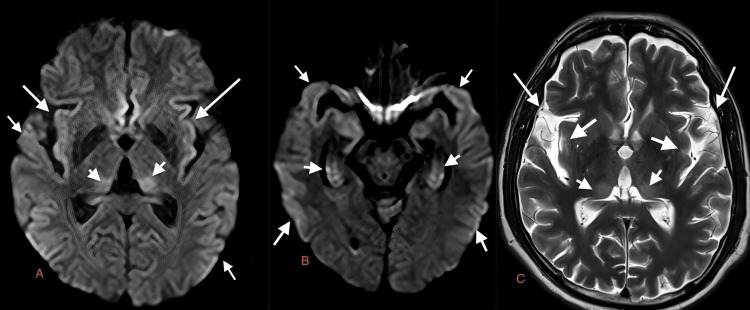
Case 6: Diffuse gyral areas of diffusion restriction are seen in both cerebral hemispheres on DWI (white arrows in A and B), predominantly in the temporal lobe also involving both hippocampi on day one of presentation in a 15-year-old male patient with dengue encephalitis. Areas of diffusion restriction are also seen in both thalami on DWI with corresponding hyperintensities on T2W images (white short arrows in A, B, and C, respectively). T2W: T2-weighted image; DWI: diffusion-weighted image
